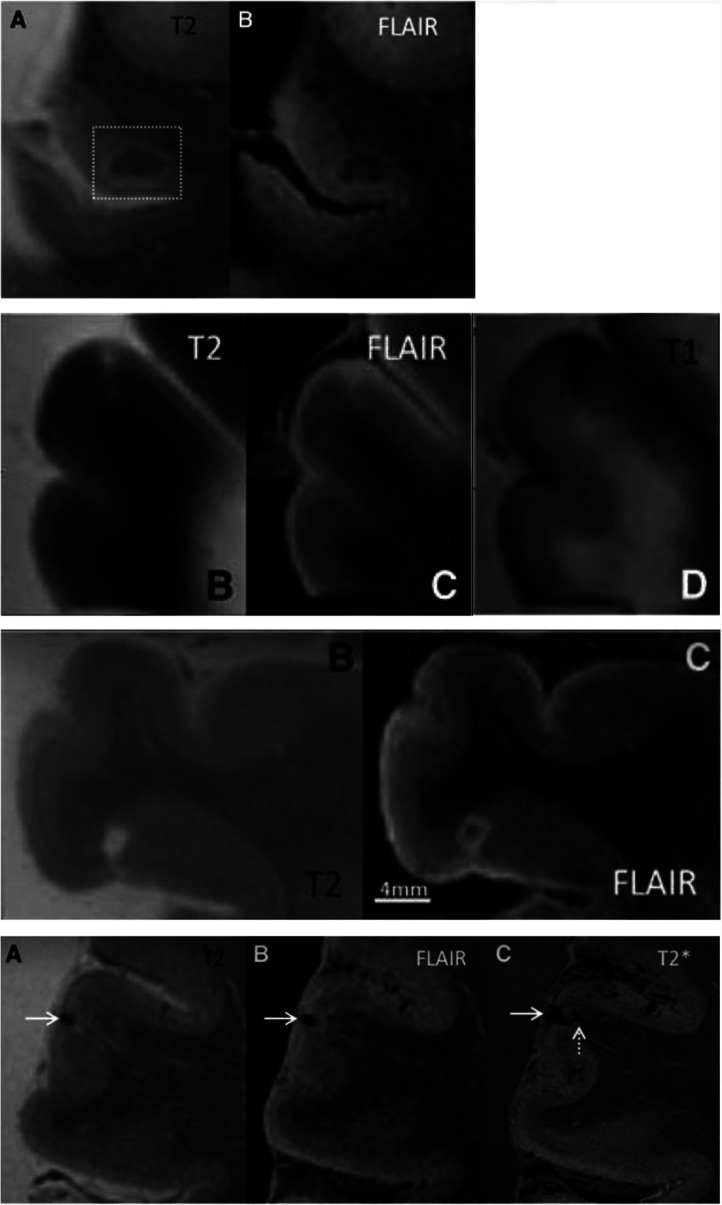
Post-mortem MR images at 7T. Row 1: Intracortical acute microinfarct has the same signal intensity as the white matter on T2 (A) and FLAIR (B). Row 2: Intracortical chronic gliotic noncavitated microinfarct appearing hyperintense on T2 (B) and FLAIR (C), and hypointense on T1 (D). Row 3: Intracortical chronic gliotic microinfarct with cavitation appearing hyperintense on T2 (B), and hypointense surrounded by a hyperintense rim on FLAIR (C). Row 4: Intracortical chronic gliotic microinfarct with hemorrhagic components (indicated by arrow) appearing hypointense on T2 (A) and FLAIR (B) and T2∗ (C) where it appears enlarged due to the blooming effect. (Courtesy of Dr. Susanne J. van Veluw, Van Veluw Lab for Neuroimaging, Massachusetts General Hospital, Boston, MA, USA).
